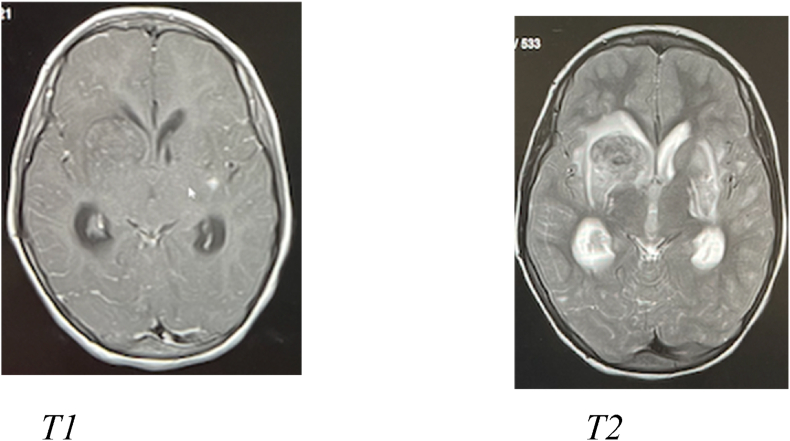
MRI study shows the hemorrhage to be isodense and intense in T1 and T2. However, it shows blooming of the gradient sequence confirming the ipsihemorrhagic nature also there are left basal ganglia hemorrhage noted in the gradient sequence. CTA of the aorta reveals mild narrowing in the distal aorta bimural thickening and apparent hypertrophy of the left ventricular wall.
